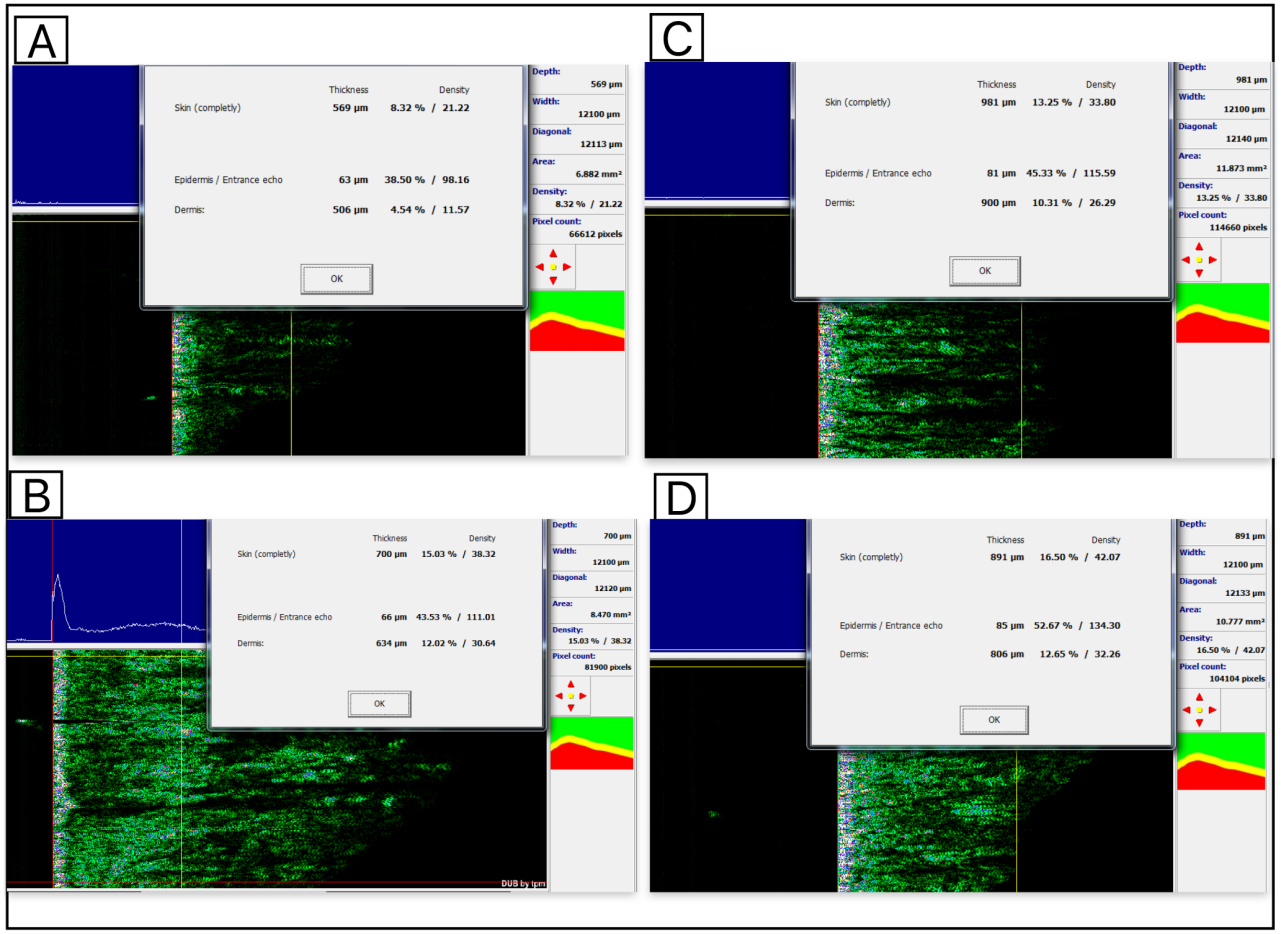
The sonographic study of a patient before and after treatment; before treatment with fibroblasts and PRP injection (A), before treatment with PRP injection (B), after treatment with fibroblasts and PRP injection (C), after treatment with PRP injection (D).
